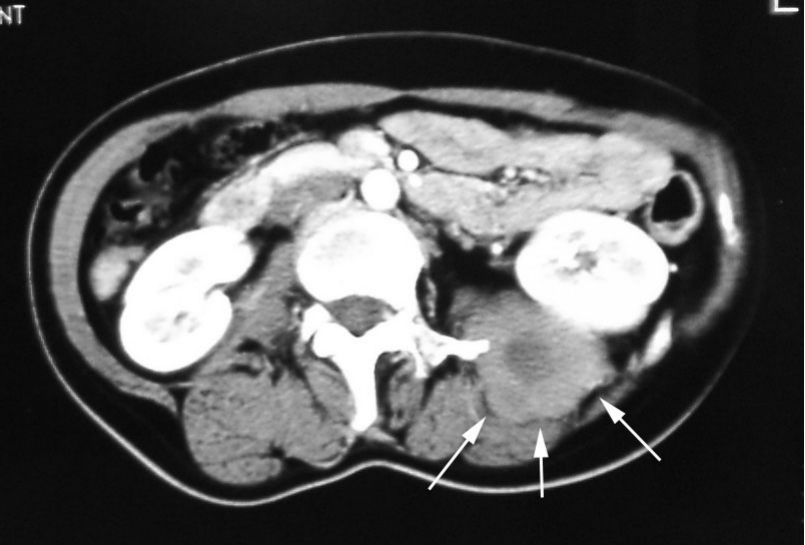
Contrast enhanced computed tomography showing the tumor location between iliocostalis and psoas muscles in retroperitoneal space.
